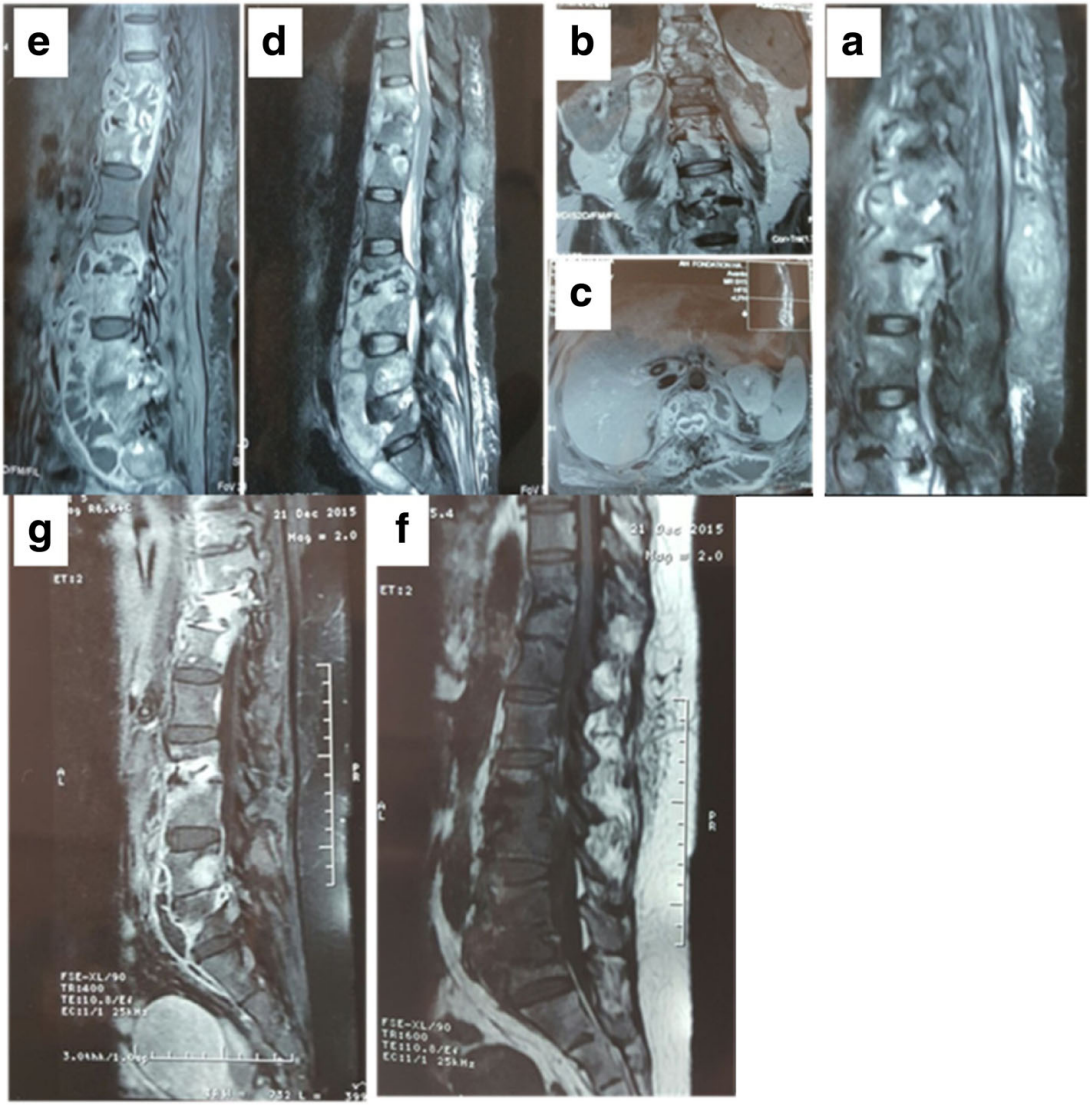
a, b, c, d and e Dorsolumbar MRI showing the evolution of the destruction and abscess of psoas and subcutaneous. f and g MRI scan after 12 months of treatment
